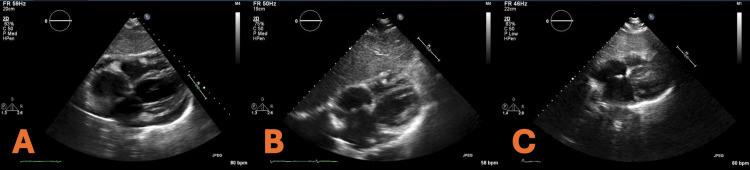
Echocardiograph from day 9 demonstrating early calcification forming on the pericardium and trivial pericardial effusion (A), echocardiograph from day 25 showing worsening calcification of the pericardium (B), and echocardiograph two months after onset of recurrent pericarditis showing resolution of the calcification of the pericardium (C).
